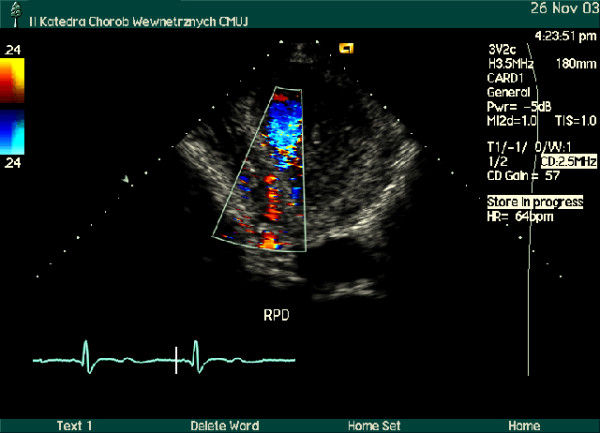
Modified apical four-chamber view, color Doppler examination. Flow detected in long segment of the right posterior descending (RPD) coronary artery is depicted in red.
